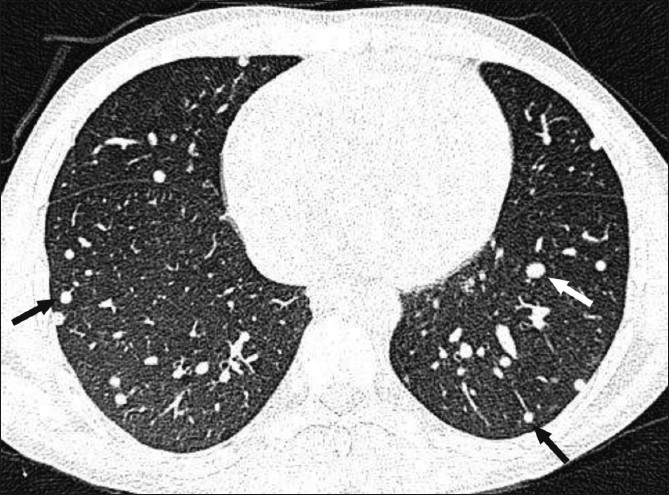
HRCT of the lungs shows multiple randomly distributed nodules (arrows) of varying sizes
